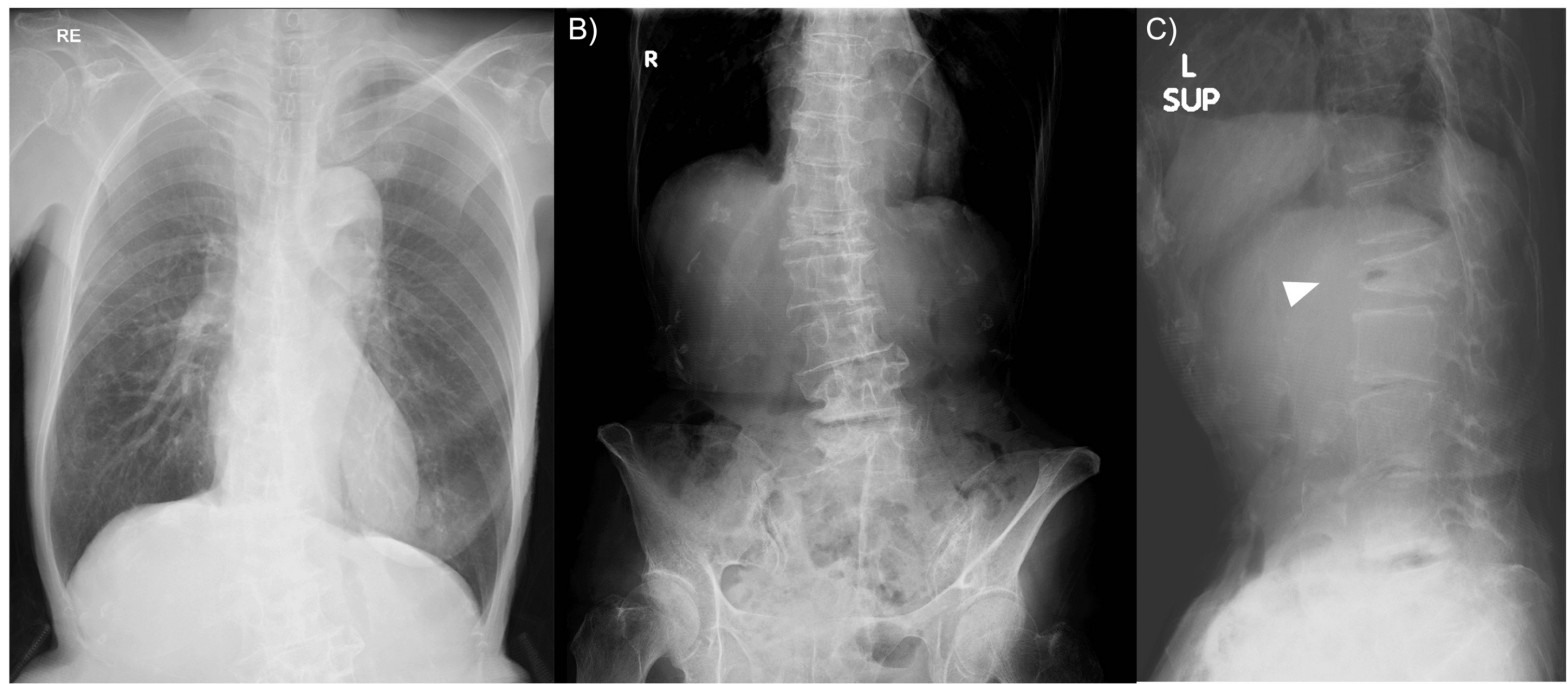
Preoperative Radiographs of the Chest and Lumbar Spine Preoperative chest radiograph (A) and lumbar spine anteroposterior (B) and supine lateral (C) radiographs showing no evidence of pneumothorax. The arrowhead in C indicates the fractured vertebra.
